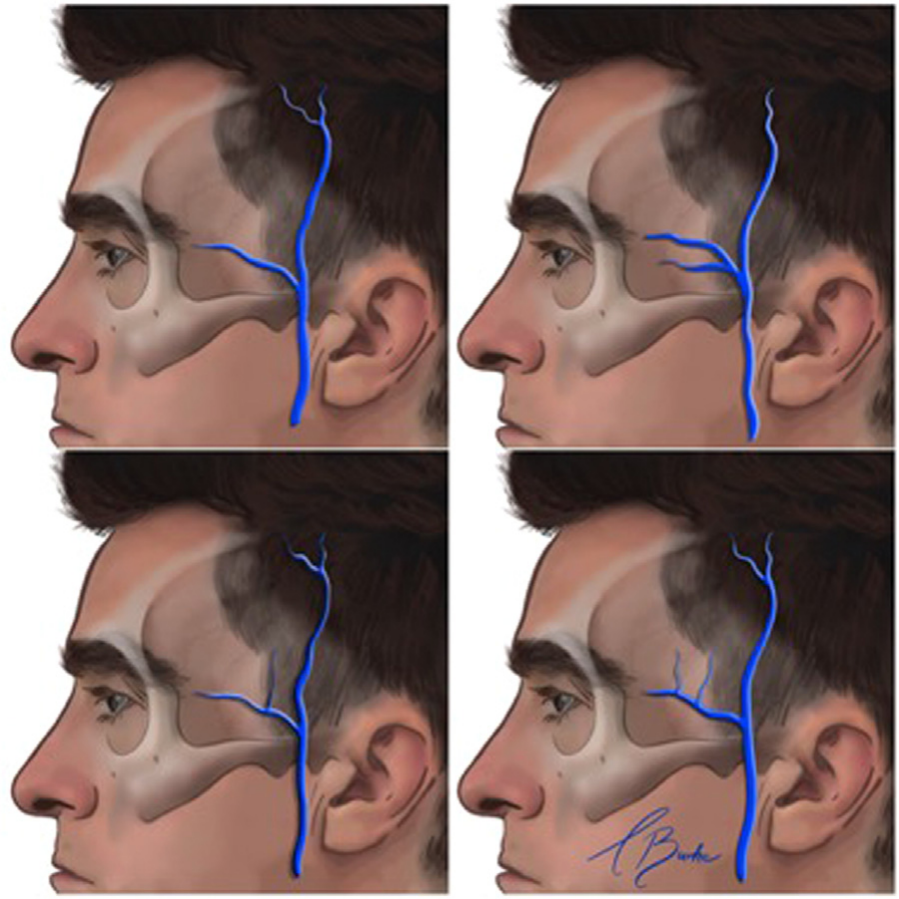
Course and branching pattern of the middle temporal vein (MTV). (Left) Only one major trunk of the MTV is present. (Right) Two major trunks of the MTV. (Lower left) One major trunk of the MTV with a small branch. (Lower right) One major trunk of the MTV with two small branches. Reproduced with permission from Wang D, Xiong S, Zeng N, Wu Y. The middle temporal vein on computed tomographic angiography: Implications for filler injection and reconstructive surgery. Plastic and Reconstructive Surgery. 2023;151(2):315–24.
